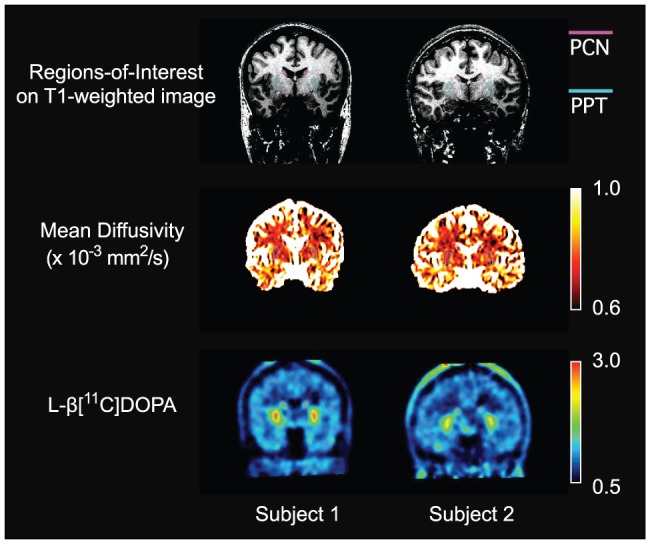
Typical images for subjects with high and low mean diffusivity (subjects 1 and 2, respectively) and corresponding mean diffusivity images. Regions of interest on T1-weighted images for posterior caudate and putamen (PCN and PPT) are also shown.
